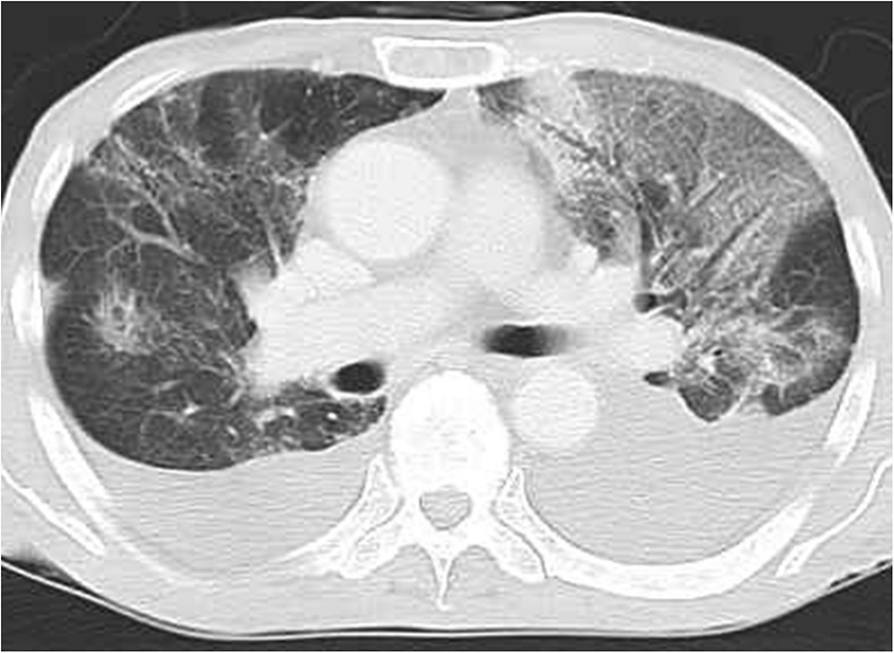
Chest computed tomography on admission. Bilateral pleural effusion and diffuse ground-glass opacity was apparent, and thickened septal lines and diffuse reticular opacity were evident in both lungs.
